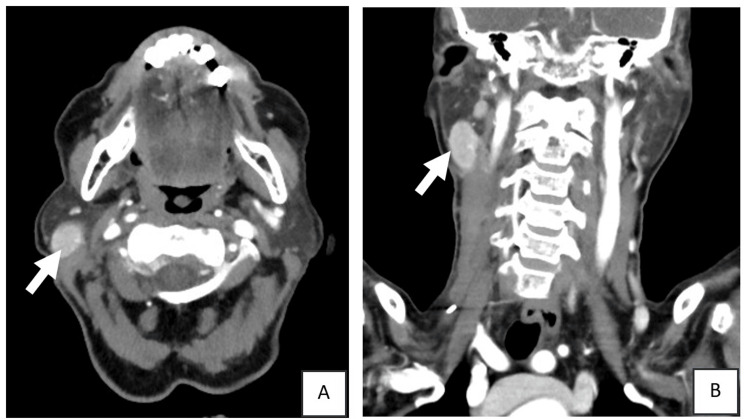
Contrast-enhanced CT images of the neck in (A) axial and (B) coronal planes demonstrate a well-circumscribed, oval-shaped, avidly enhancing soft-tissue lesion located within the right parotid gland (white arrows). The lesion shows homogeneous enhancement without central necrosis or calcification. No surrounding fat stranding or invasive features are seen. These imaging features are compatible with a benign parotid lesion.
